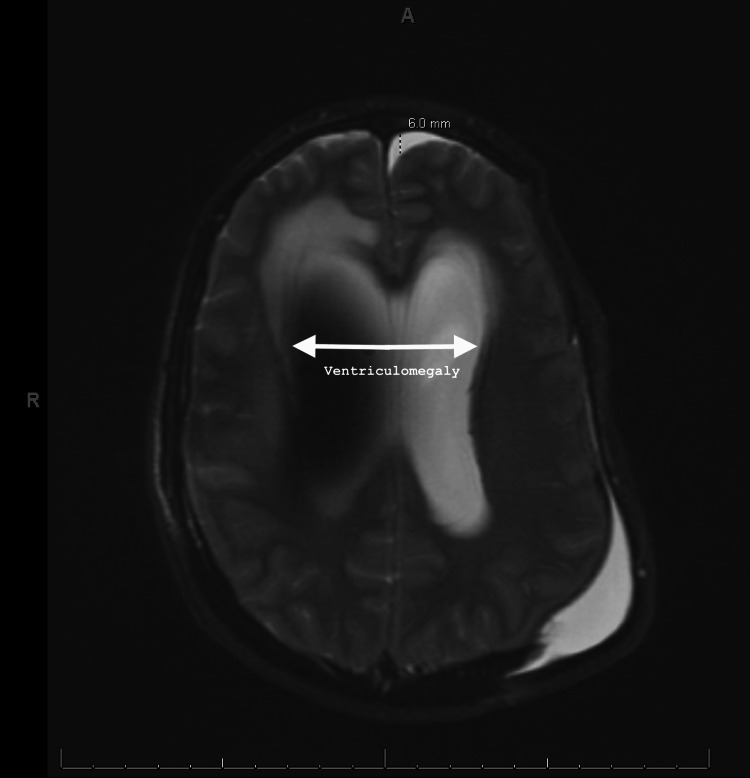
Axial T2-weighted magnetic resonance imaging (MRI) of the brain obtained on hospital day 3 demonstrating persistent severe ventriculomegaly (white arrows) with transependymal edema despite appropriate ventricular catheter positioning, suggesting impaired cerebrospinal fluid diversion.
